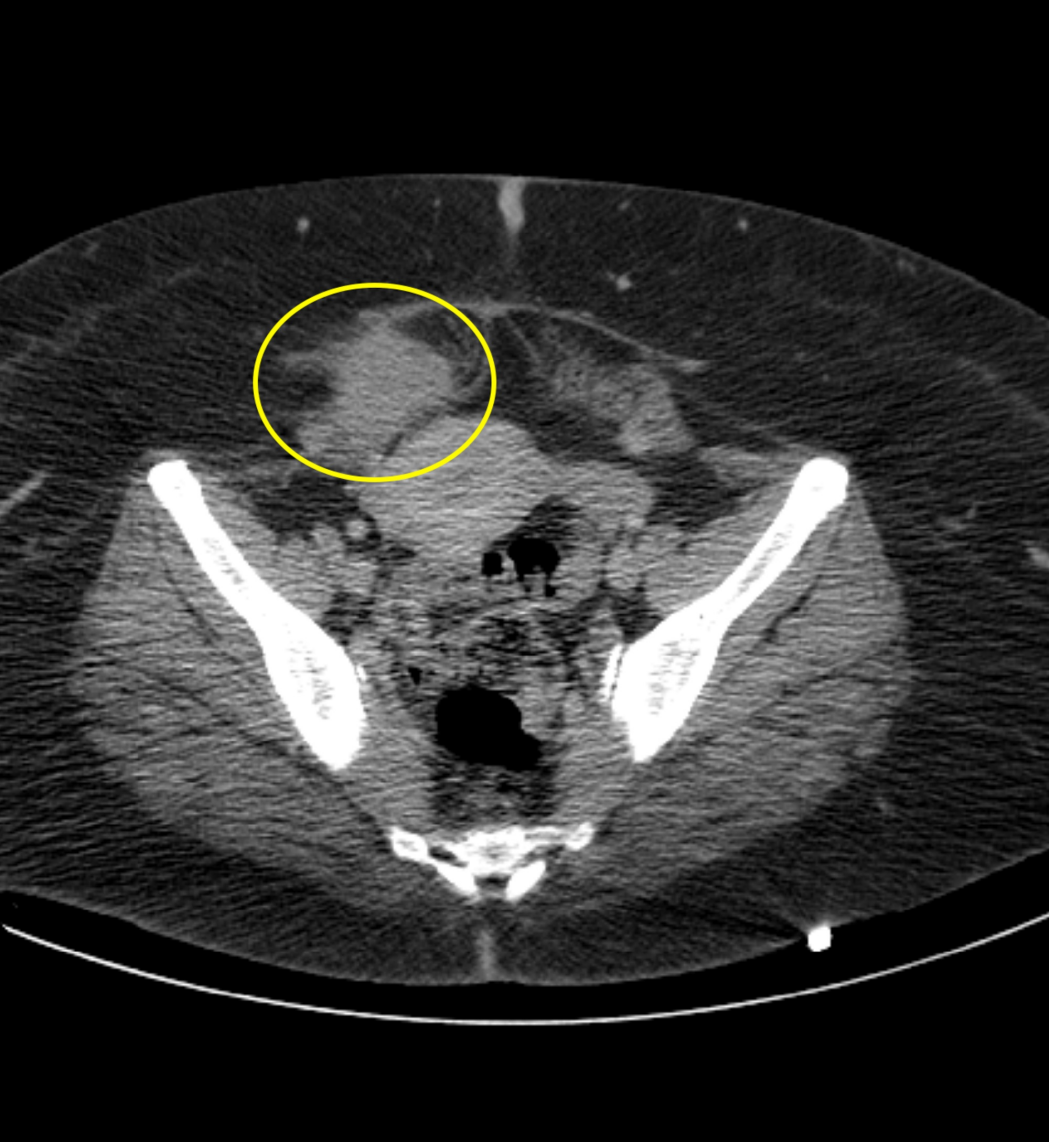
Initial emergency department CT axial demonstrates an ill-defined hyperattenuating soft tissue mass in the inferior right rectus abdominis muscle measuring approximately 5 x 4 cm with surrounding fat stranding and soft tissue edema that is consistent with an abdominal wall endometrioma (yellow circle).
